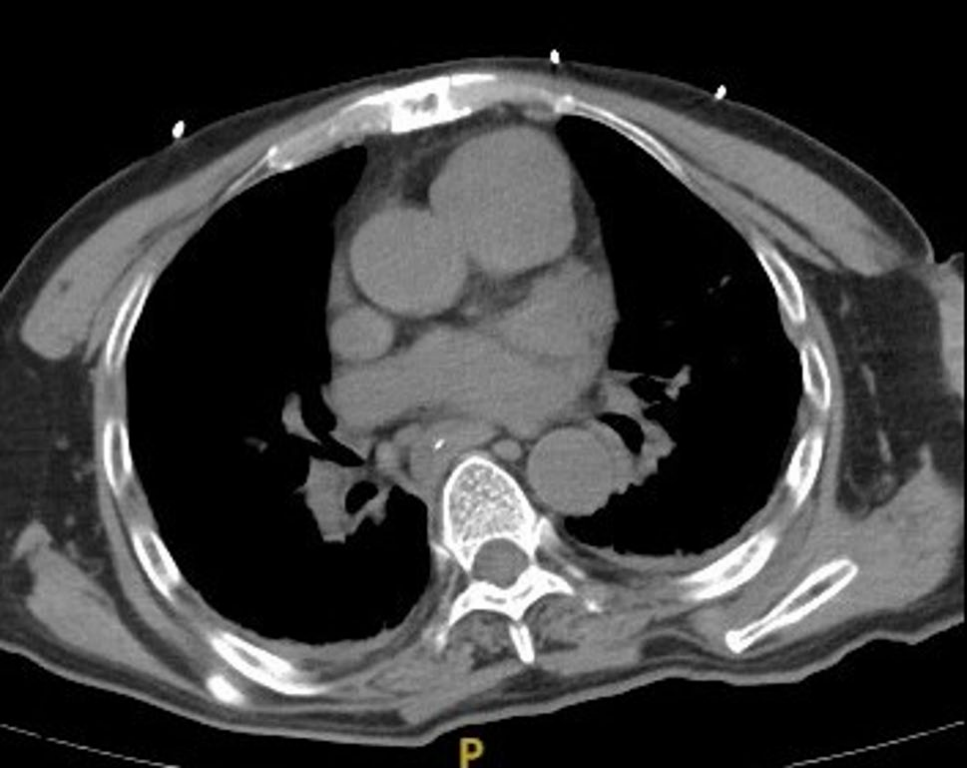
The preoperative CT showed no internal or external pericardial effusion
